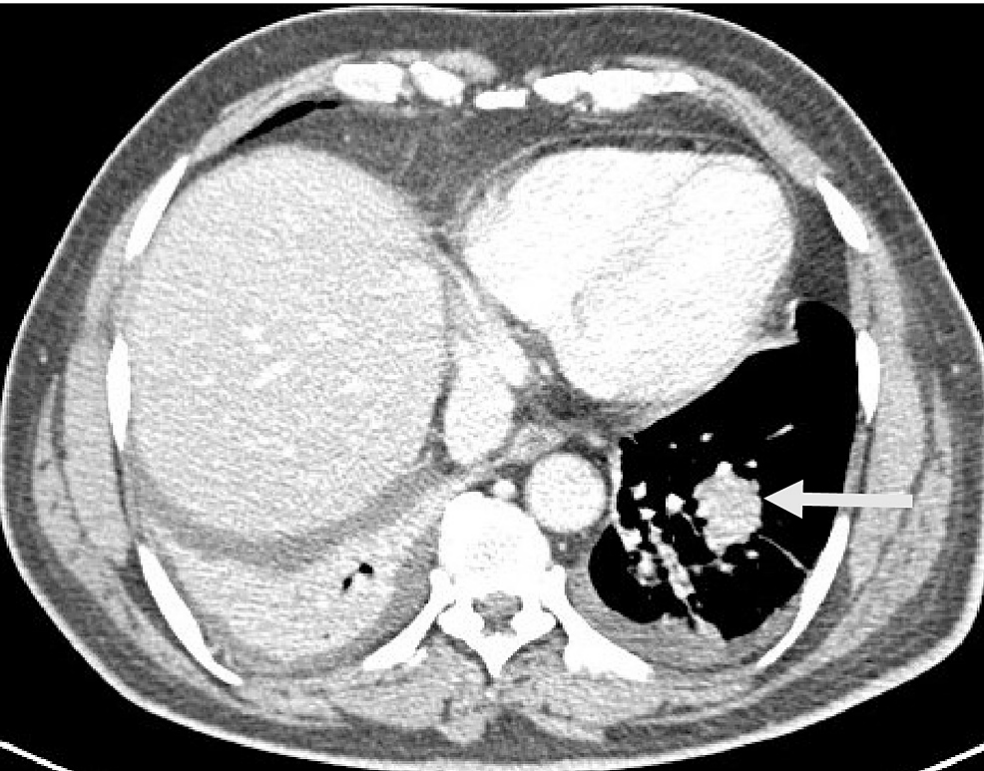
CT scan chest of the same patient’s basal cut lung window showing a left side lung mass (arrow) 2.5 cm and related atelectatic plates.
